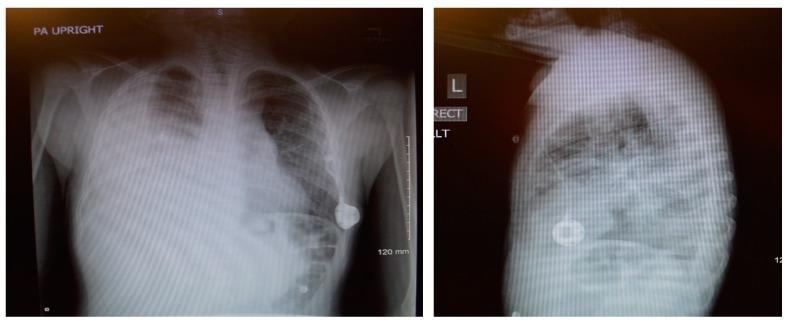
Chest X-rays demonstrating undrained right chylothorax.
